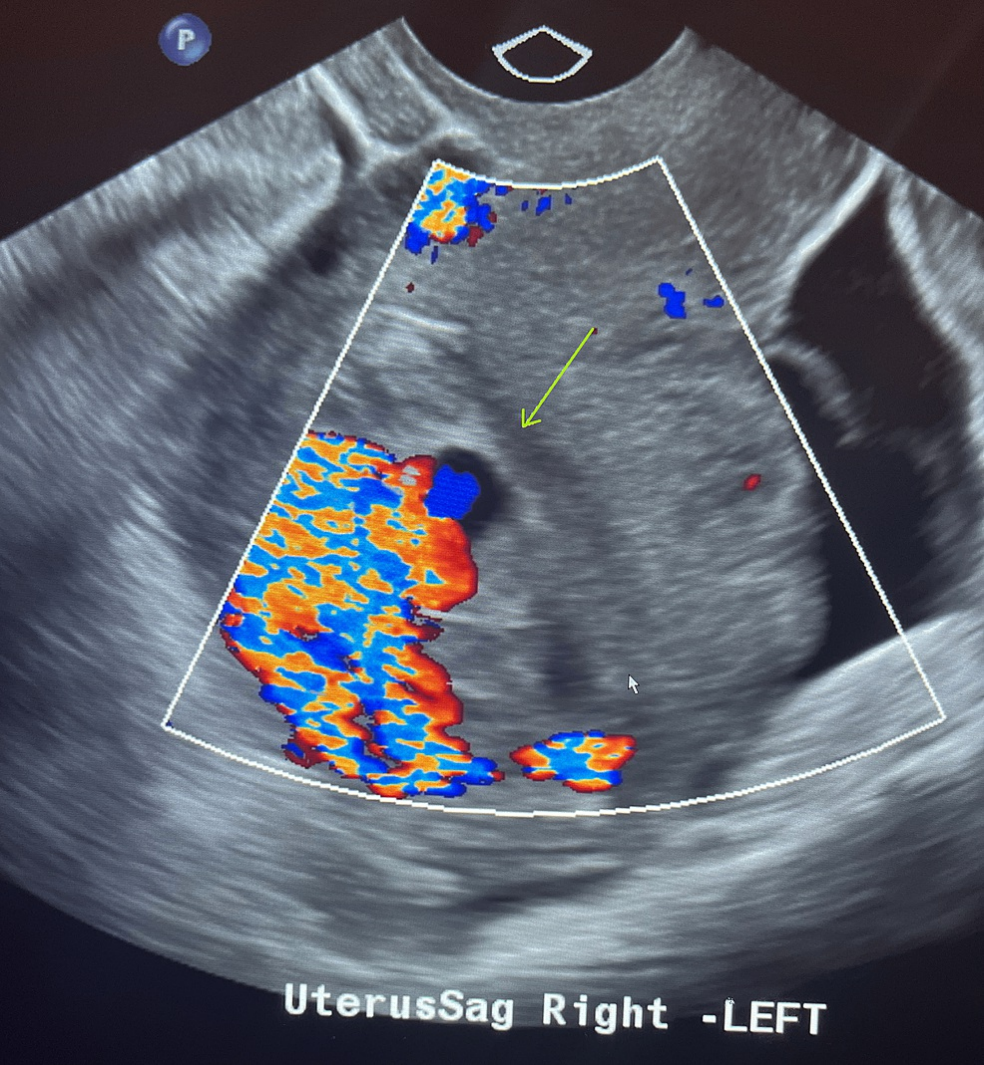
Ultrasound with Doppler. The arrow is pointing towards the endometrium, which displays vibrant abnormal flow on color Doppler.
